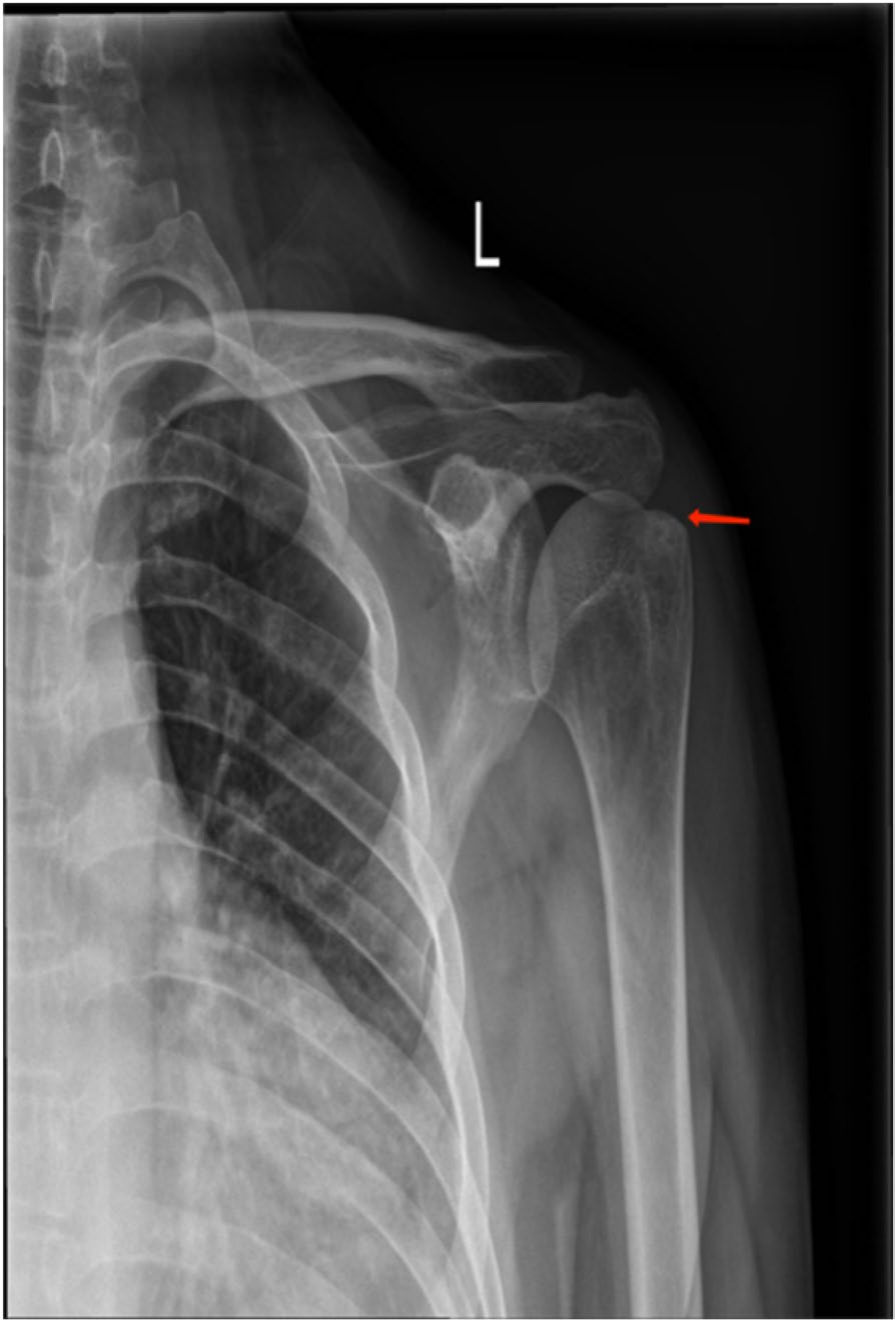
X-ray of the left humerus. Male, 50 years old
